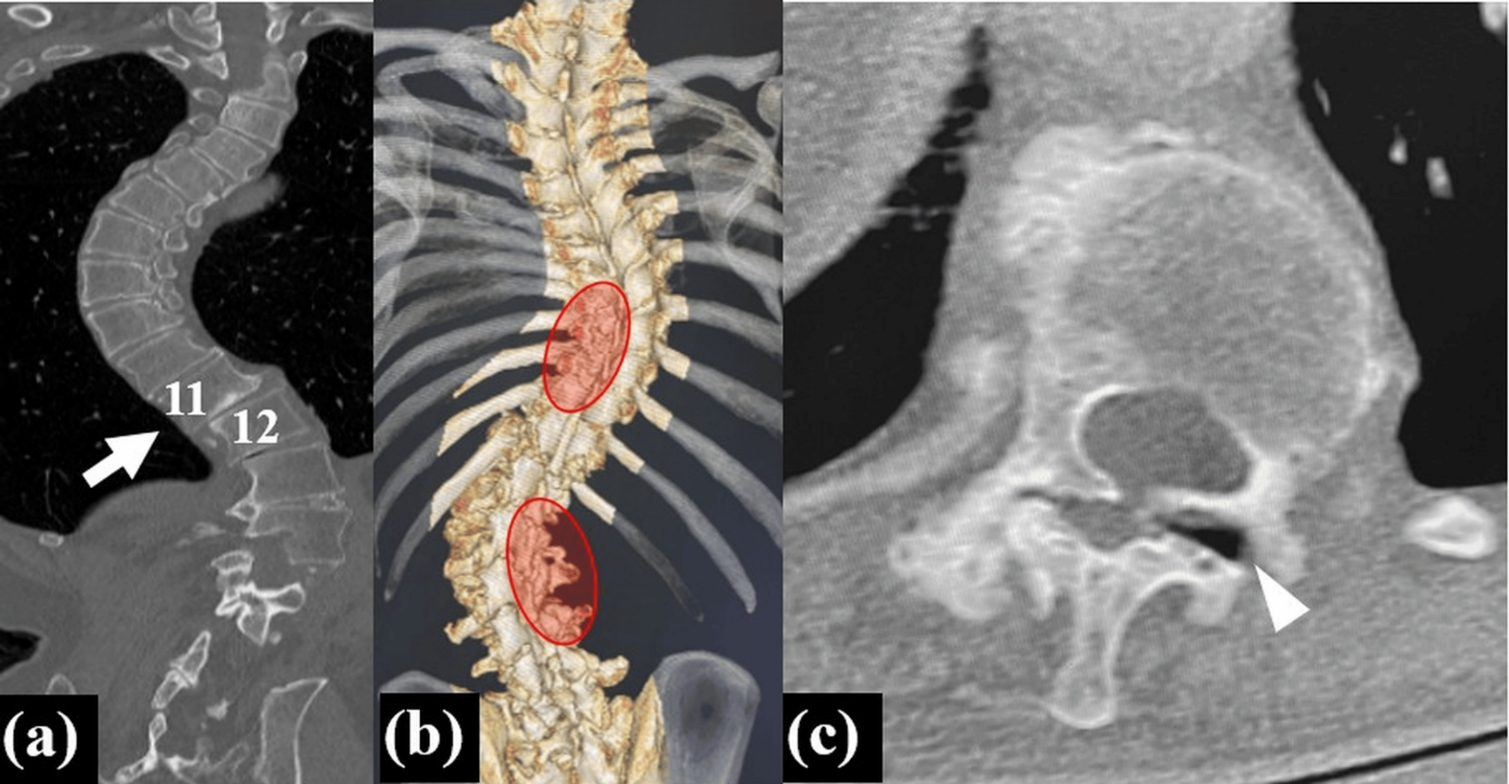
Preoperative computed tomography (CT) (a) Coronal CT showing lateral slip between T11 and T12 (white arrow). (b) Three-dimensional CT demonstrating concave-side facet fusion between T8-11 and L1-4. (c) Axial CT showing bilateral facet osteoarthritis and left T11/12 facet opening (white arrowhead).
